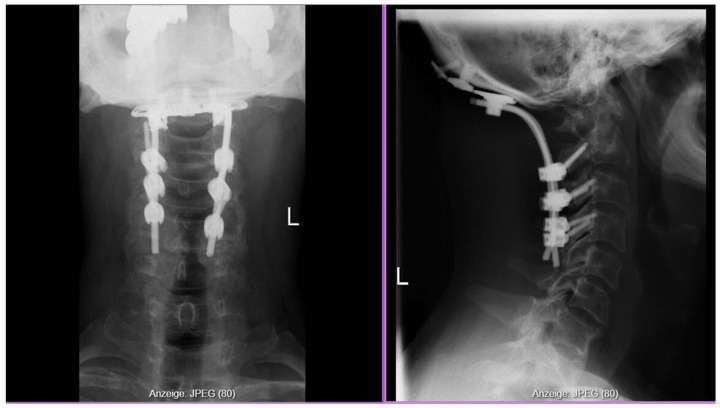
Postoperative X-ray after occipitocervical fusion (C0–C4).
